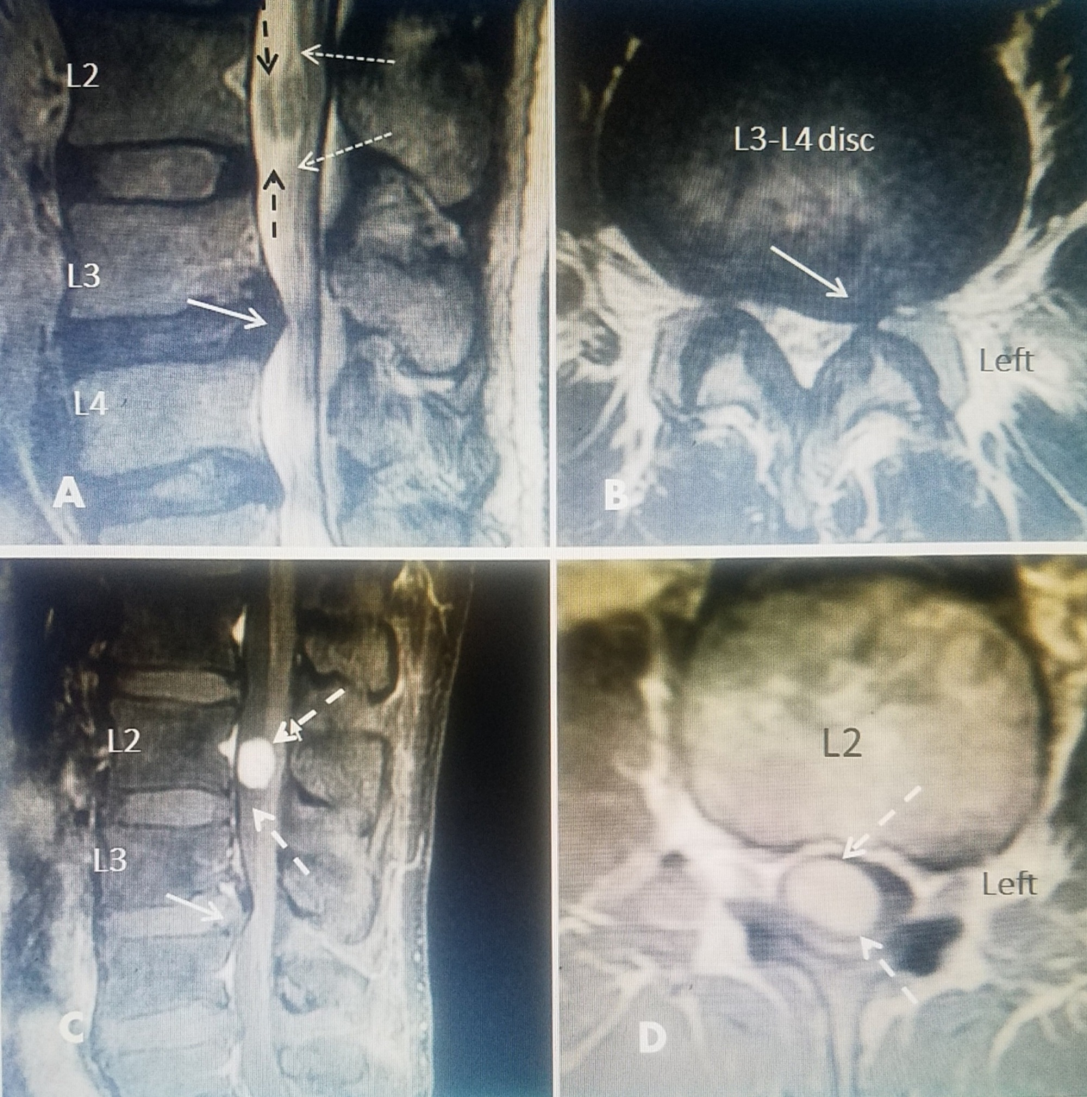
Initial and post-gadolinium (Ga+) magnetic resonance imaging (MRI). A 38-year-old male in previous good health had a minor auto accident. He originally had back pain and then developed tingling and pain down the left leg. No neurologic findings including normal reflex and motor strength. A: Sagittal T2 image showing a definite herniated L3-4 disc (solid white arrow). Report specifically stated L2 normal but there is an intradural oval lesion (dashed black arrows) with apparent dorsal displacement of cauda equina roots (dotted white arrows). B: There was no axial image through L2. Axial image at L3-4 demonstrates a left lateral herniation with foraminal narrowing (solid white arrow). C: A gadolinium (Ga^+^) MRI was performed and the large smooth enhancing lesion at the center of L2 was identified (dashed white arrow). D: Axial Ga^+^ MRI clearly shows the tumor is intradural filling 80% of the canal and more to the right side.
